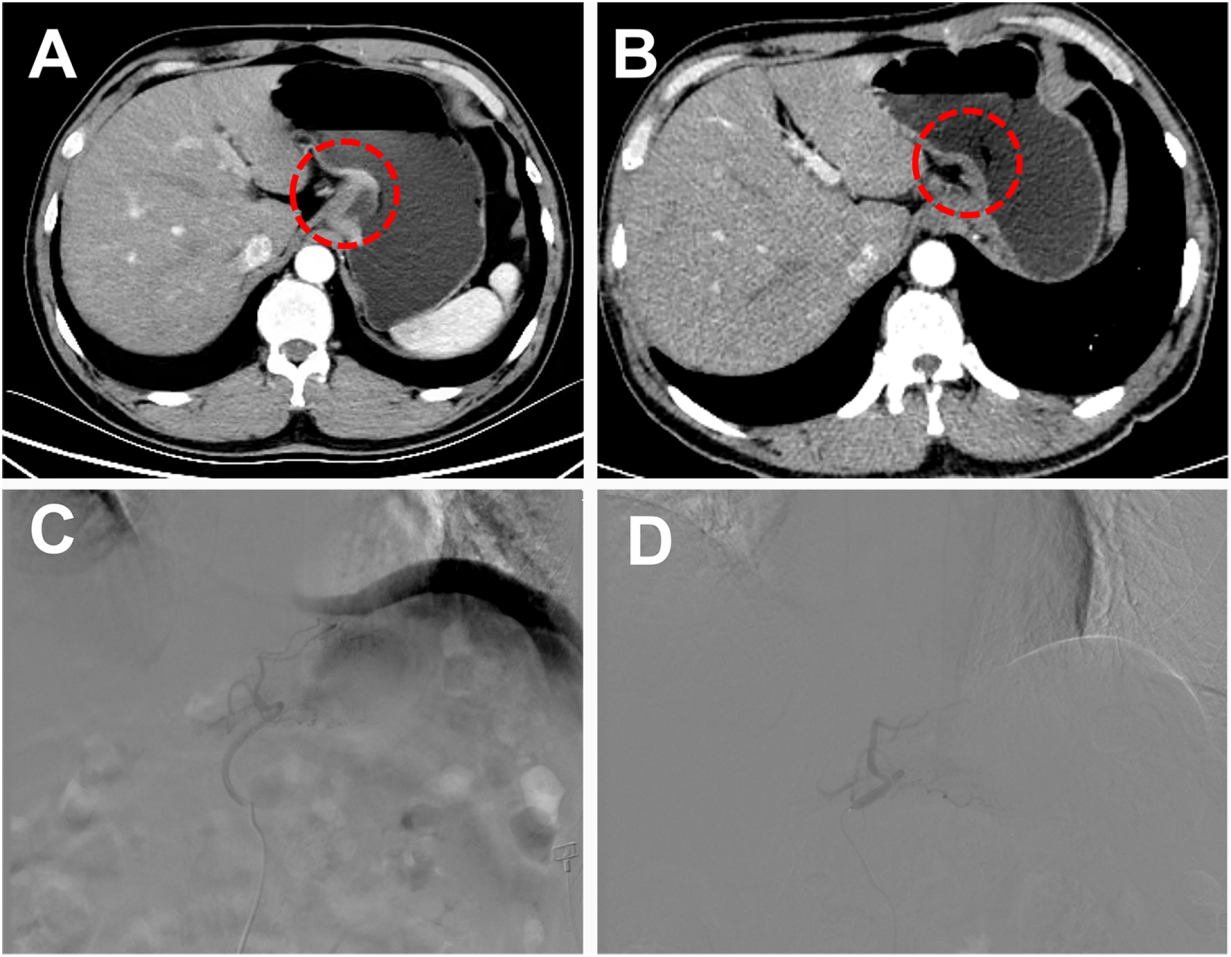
A 51-year-old male patient with low-grade adenocarcinoma at the gastroesophageal junction was offered a curative surgical opportunity after TACE combined with targeted drugs and immunotherapy. (A) Abdominal Computer Tomography (CT) showed a tumor at the gastroesophageal junction during the arterial phase before combined treatment. (B) After 4 months of combined treatment, the patient’s abdominal CT arterial phase enhancement of the original tumor disappeared. (C) Under the guidance of digital subtraction angiography (DSA), the tip of the 5-F catheter with guidewire was introduced to the aorta, and select the left gastric artery to perform angiography and observed the blush of the tumor. (D) The operator slowly injected the iodized oil-oxaliplatin emulsion through the microcatheter until the tumor blush disappeared.
